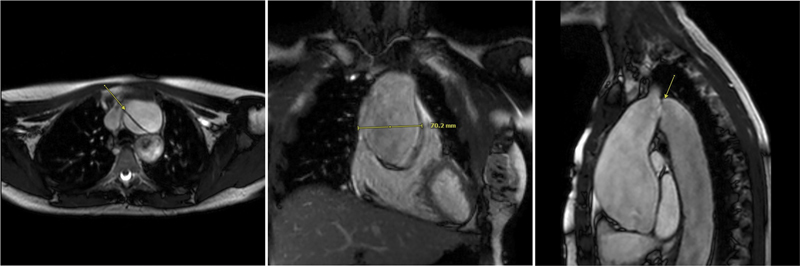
MRI images of Sibling A. Left: Axial view showing an aortic dissection flap. Middle: Coronal view demonstrating significant aortic dilation. Right: Sagittal view revealing paraductal pseudocoarctation. MRI, magnetic resonance imaging.
